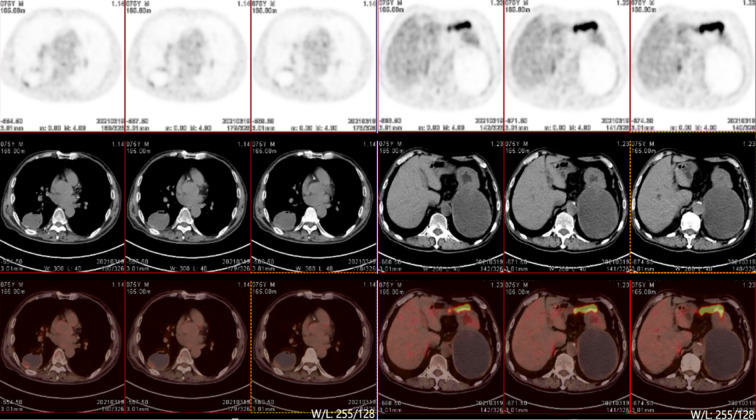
PET/CT scan results of the patient.
